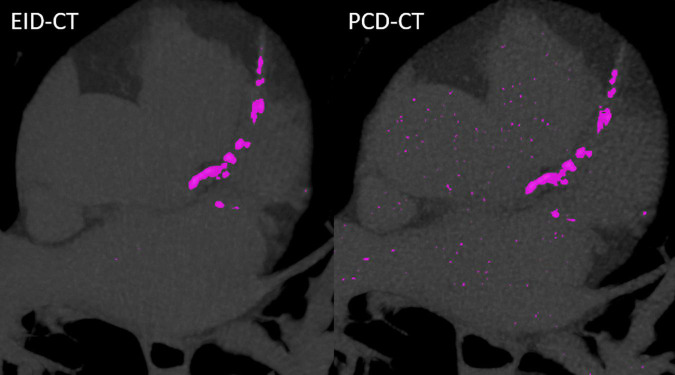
Case example from a patient scanned in the EID-CT (CACS_*EID–CT*_: 964.1) and PCD-CT (CACS_*PCD–CT*_: 949.9).
